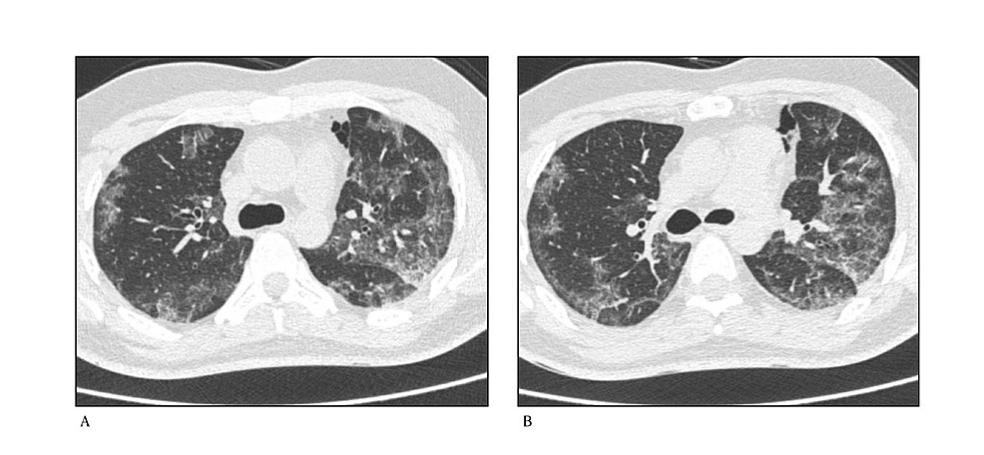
HRCT Chest lung window axial sections A) and B) Patchy multifocal (both peripheral and central distribution) ground glass opacities with areas of associated inter/intralobular septal thickening giving crazy paving pattern
